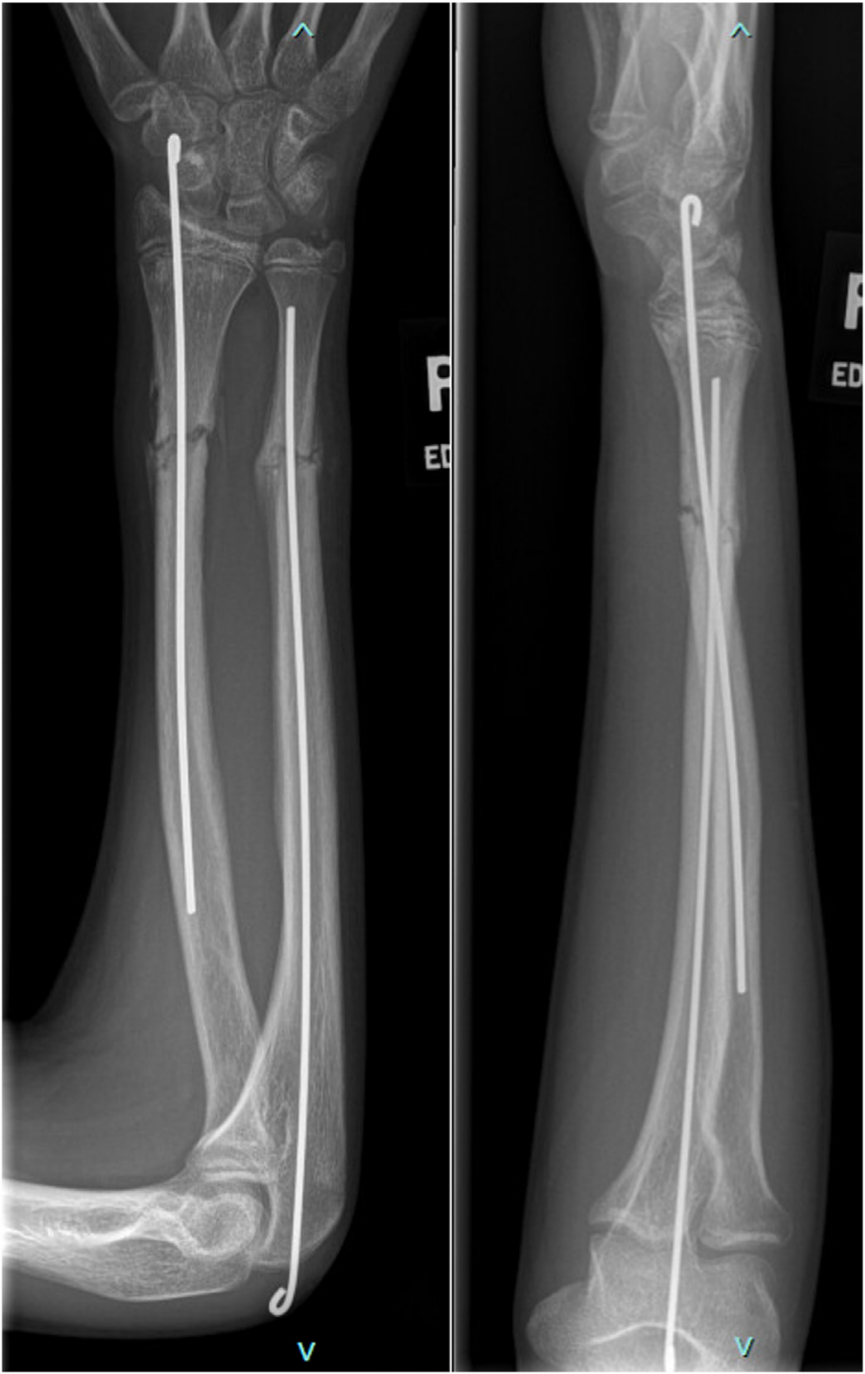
Lateral and poster–anterior radiographs taken after surgery demonstrating reduction of the fracture with flexible intramedullary nails.
